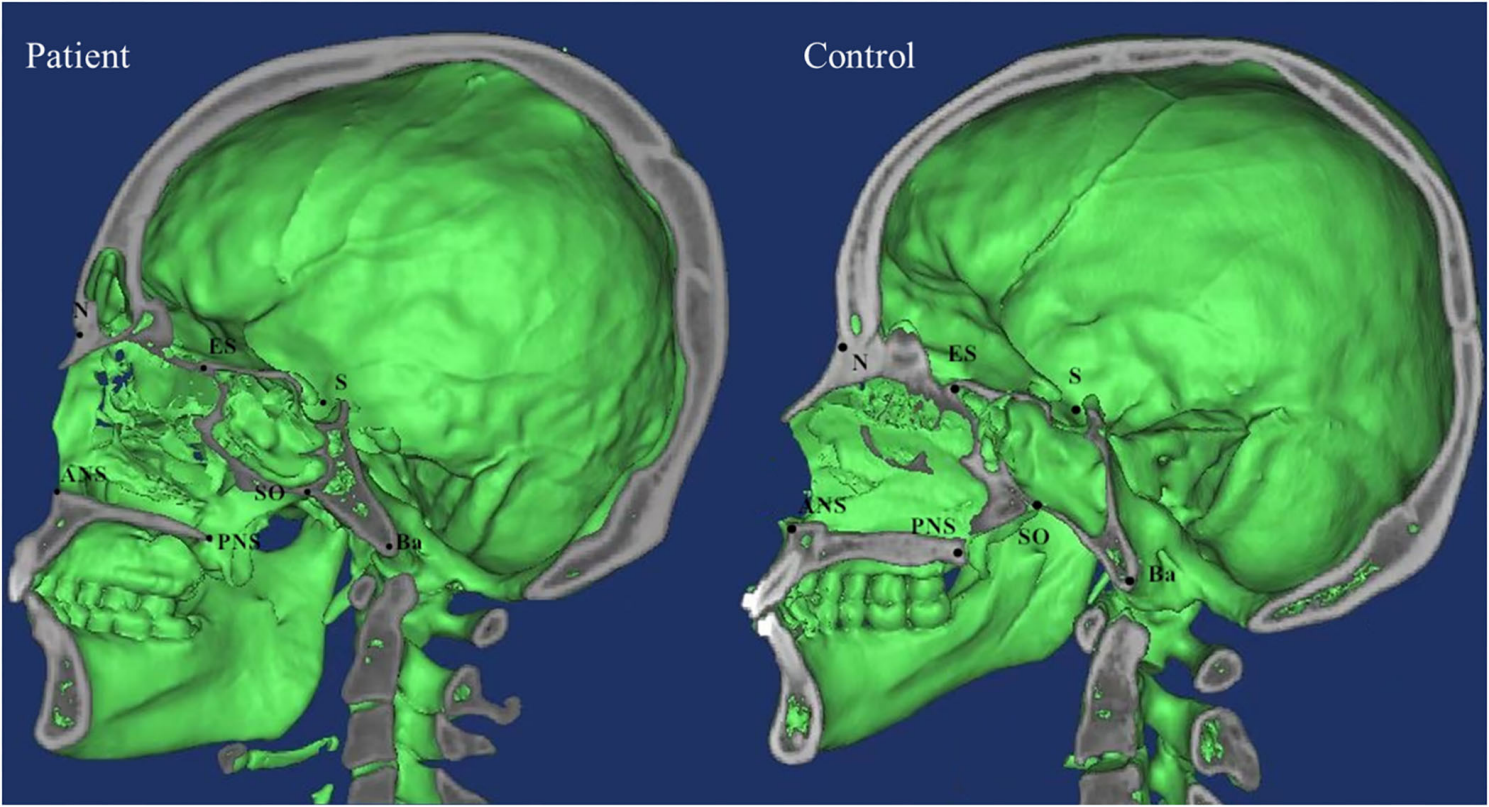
The anatomical landmarks were shown on the computed tomographic scans (sagittal scan).
